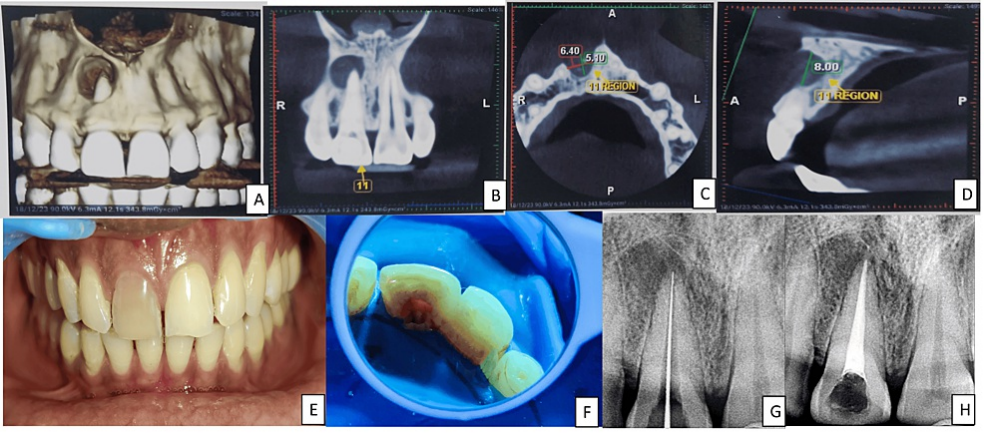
(A) CBCT with tooth number 11, (B) coronal section with tooth number 11 showing large periapical lesion, (C) axial section with tooth number 11 showing the extent of the lesion, (D) sagital section with tooth number 11 showing the lesion diameter of 8 mm, (E) intraoral picture showing discoloration with tooth number 11, (F) access opening with tooth number 11, (G) RVG of working length with tooth number 11, (H) RVG of obturation with tooth number 11 CBCT: Cone-beam computed tomography; RVG: radiovisiography
